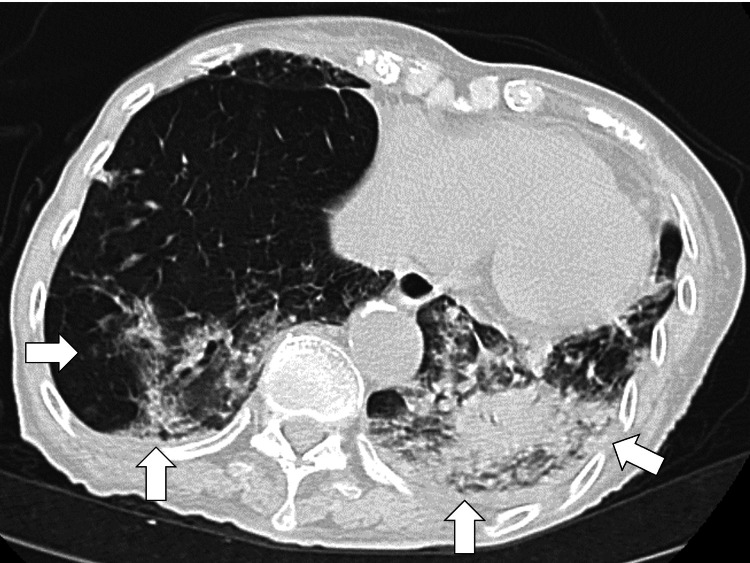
Chest CT revealing a scar-like lesion, granular shadows in the left lung, poorly defined infiltrates, and reduced lung transparency (white arrows) CT: computed tomography
